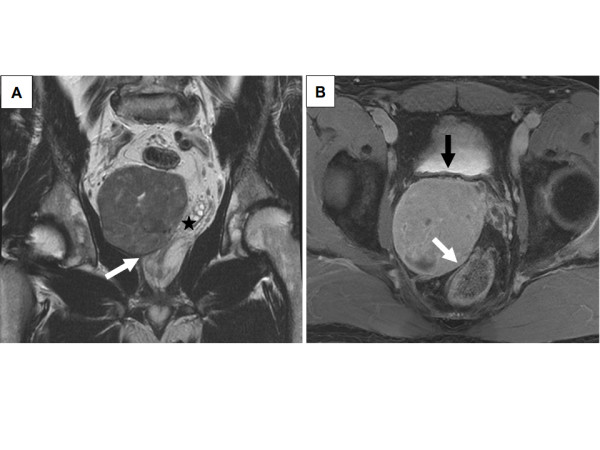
Magnetic resonance imaging of the pelvis. A/Coronal T2-weighted MRI showing an heterogeneous mass lesion in the region of right seminal vesicle, causing inferiorly and to the left displacement of the prostate (white arrow) and of the left seminal vesicle which appears normal (star). B/Axial contrast-enhanced T1-weighted MRI showing distinct margins between the mass and both the posterior wall of the bladder (black arrow) and the anterior wall of the rectum (white arrow).
